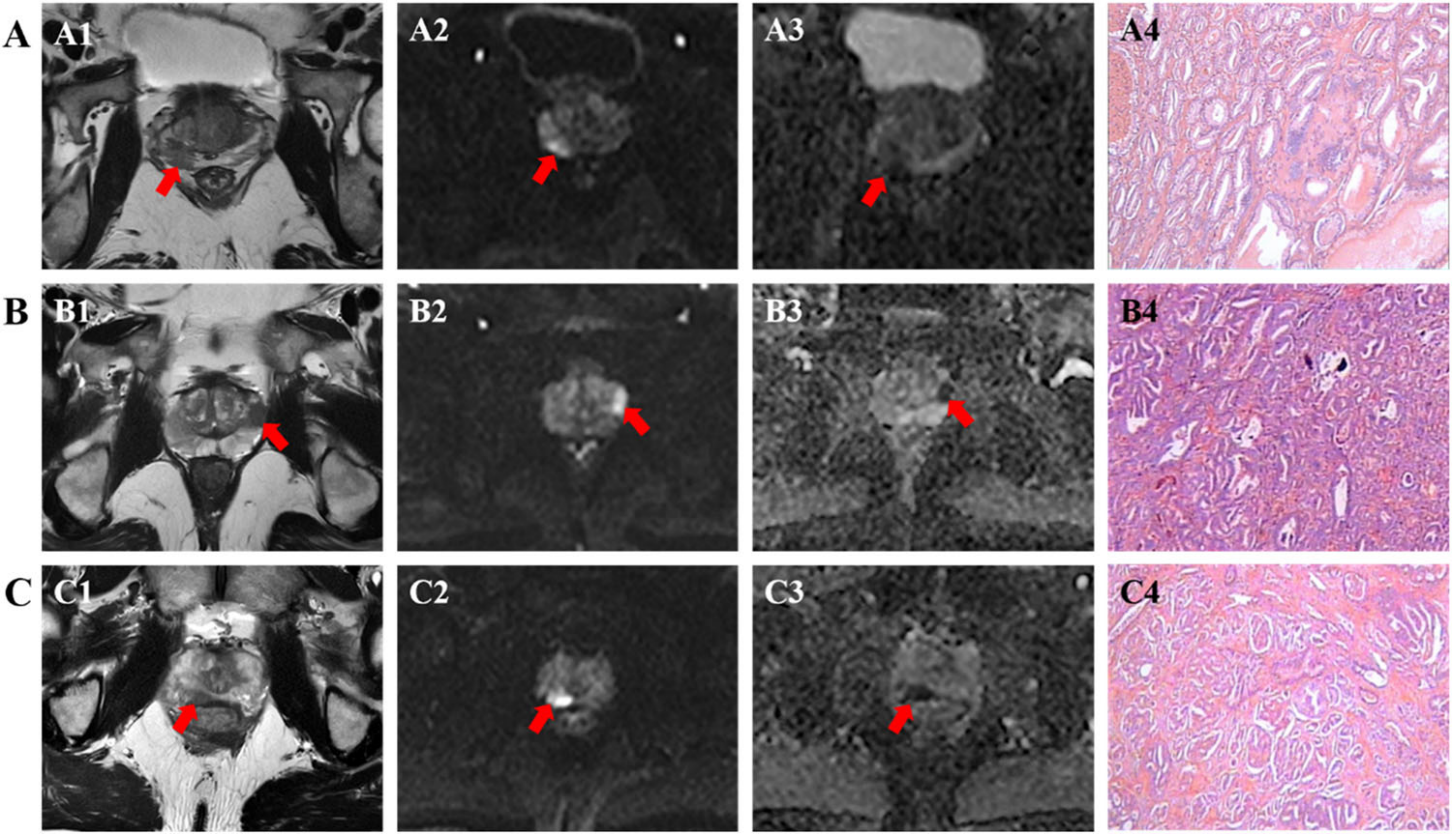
T_2_WI shows low signal (A1, B1, C1, red arrows), DWI shows high signal (A2, B2, C2, red arrows) and ADC shows low signal (A3, B3, C3, red arrows) in peripheral zone. A A 68-year-old man, PSA = 11 ng/mL, ADC_min_ = 0.576 × 10^−3^ mm^2^/s (*b* = 800), ADC_mean_ = 0.984 × 10^−^^3^ mm^2^/s (*b* = 800); GS 3 + 3 (HE × 40) (A4). B 67-year-old man, PSA = 7.68 ng/mL, ADC_min_ = 0.411 × 10^−^^3^ mm^2^/s (*b* = 800), ADC_mean_ = 0.779 × 10^−^^3^ mm^2^/s (*b* = 800); GS 3 + 4 (HE × 40) (B4). C A 72-year-old man, PSA = 6.4 ng/mL, ADC_min_ = 0.071 × 10^−3^ mm^2^/s (*b* = 800), ADC_mean_ = 0.748 × 10^−^^3^ mm^2^/s (*b* = 800); GS 4 + 3 (HE × 40) (C4)
